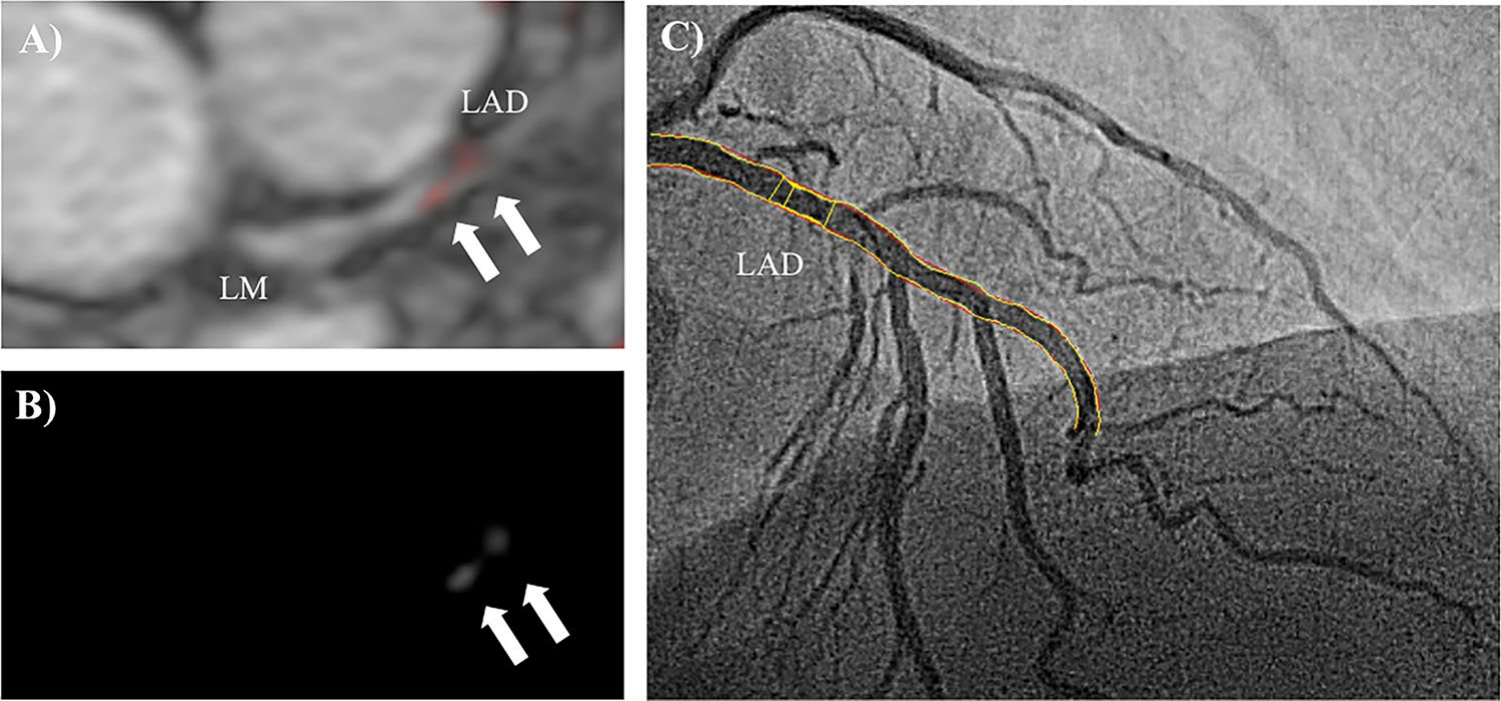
Representative images demonstrating on minimal signal enhancement at sites of non-relevant stenosis following the administration of an albumin-binding MR probe. To highlight the anatomical relationship between the uptake of the albumin-binding-MR probe (b) and morphology from the MR angiography, images were fused in a way comparable to positron emission tomography/computed tomography (a). Subsequent Invasive catheterization demonstrated only a minimal stenosis in the left arterial descending artery (LAD) (C)
